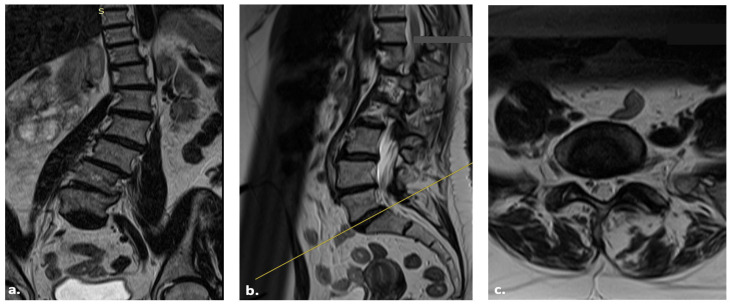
Coronal (a), sagittal (b) and axial (c) MRI scans of the patient showing the coronal and sagittal imbalance.
